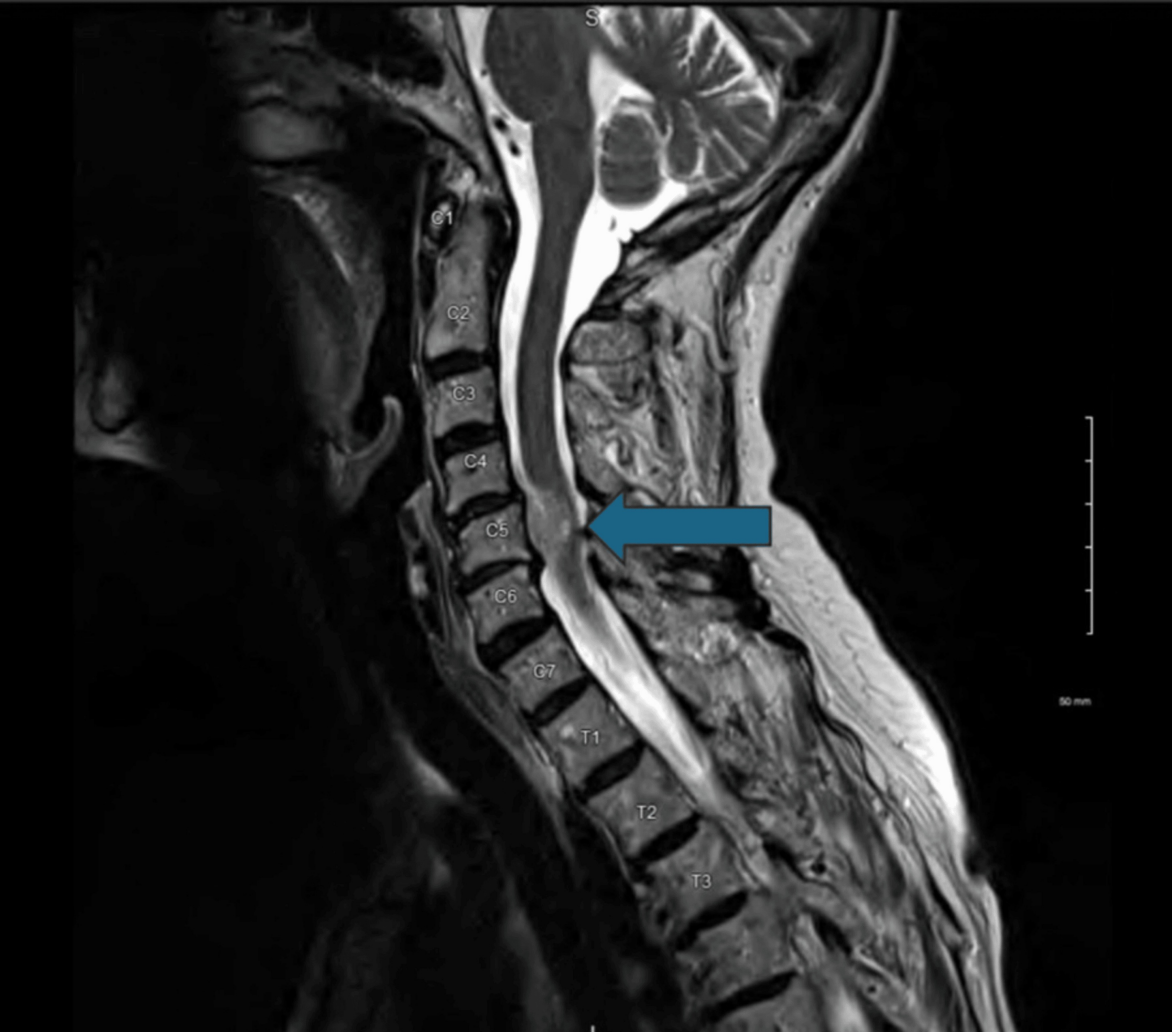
T2-weighted magnetic resonance imaging scan in the sagittal plane showing the suspected meningioma (blue arrow).
